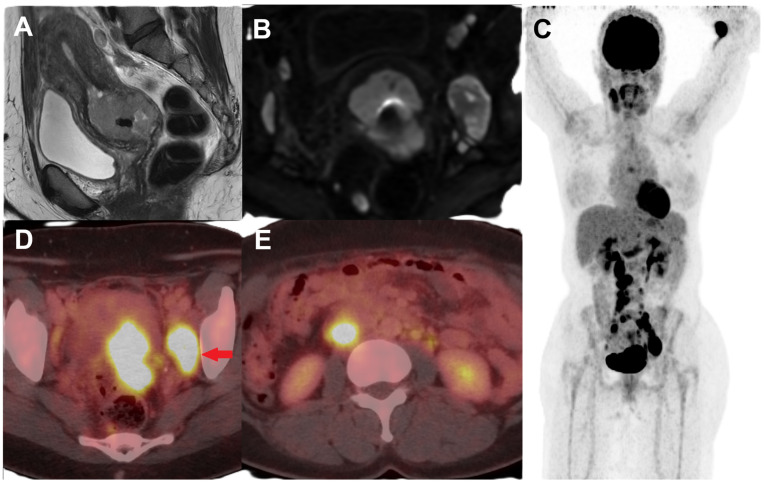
Female with newly diagnosed HPV-associated squamous cell cervical cancer, Stage IIIC. T2-weighted sagittal MR (A) and diffusion-weighted (B) images show a circumferential mass involving the cervix, extending into the lower uterine segment, and suspicious parametrial invasion in the left posterolateral region as well as enlarged pelvic lymph nodes. (C) Maximum intensity projection (MIP) image of FDG PET/CT performed for initial staging showing FDG-avid primary cervical lesion with metastatic FDG-avid enlarged left obturator node ((D), red arrow) and FDG-avid retroperitoneal metastatic lymph nodes (E).
